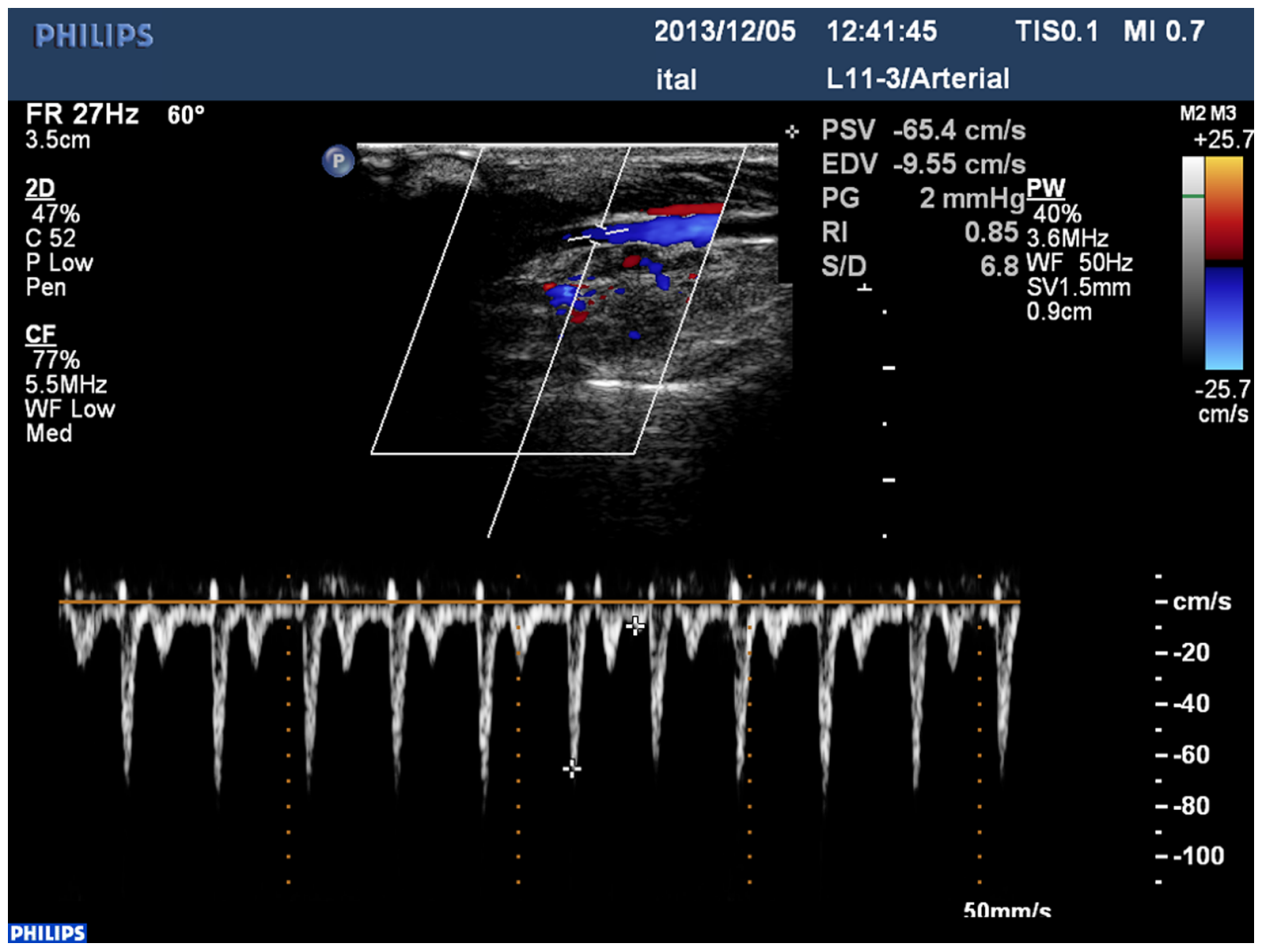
Results determined by color Doppler ultrasound.
